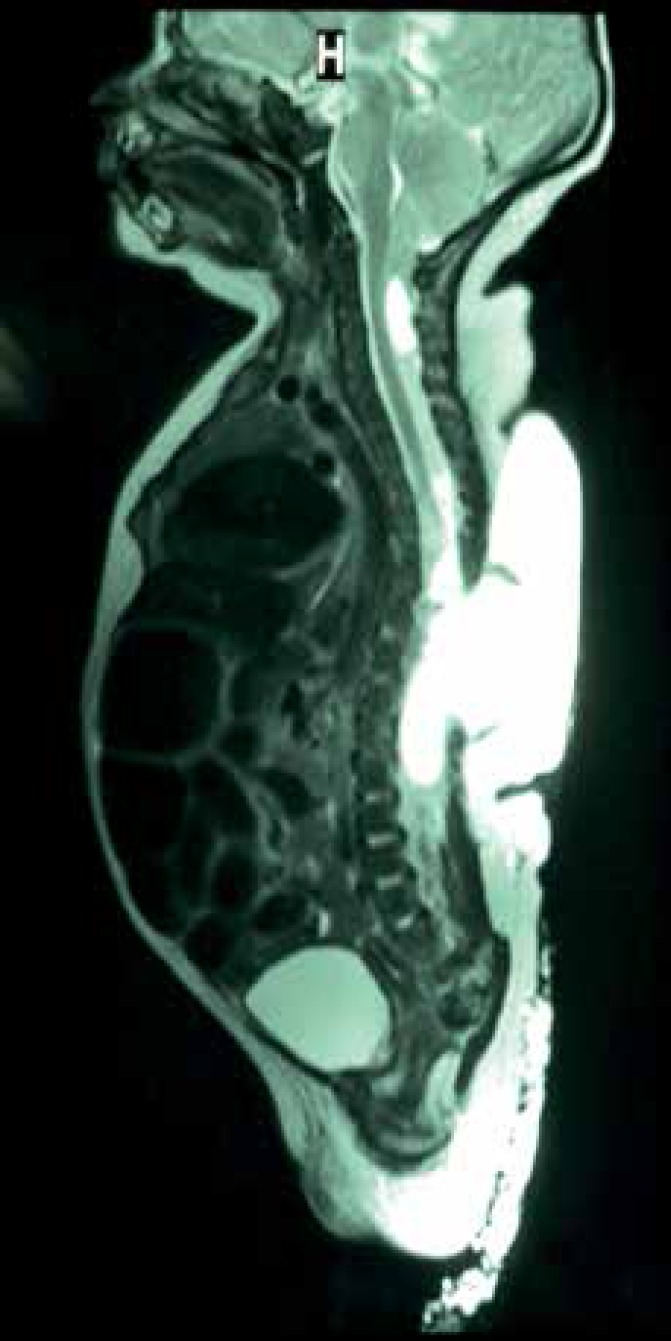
MRI showing cerebellar displacement with tonsillar herniation of 12mm
